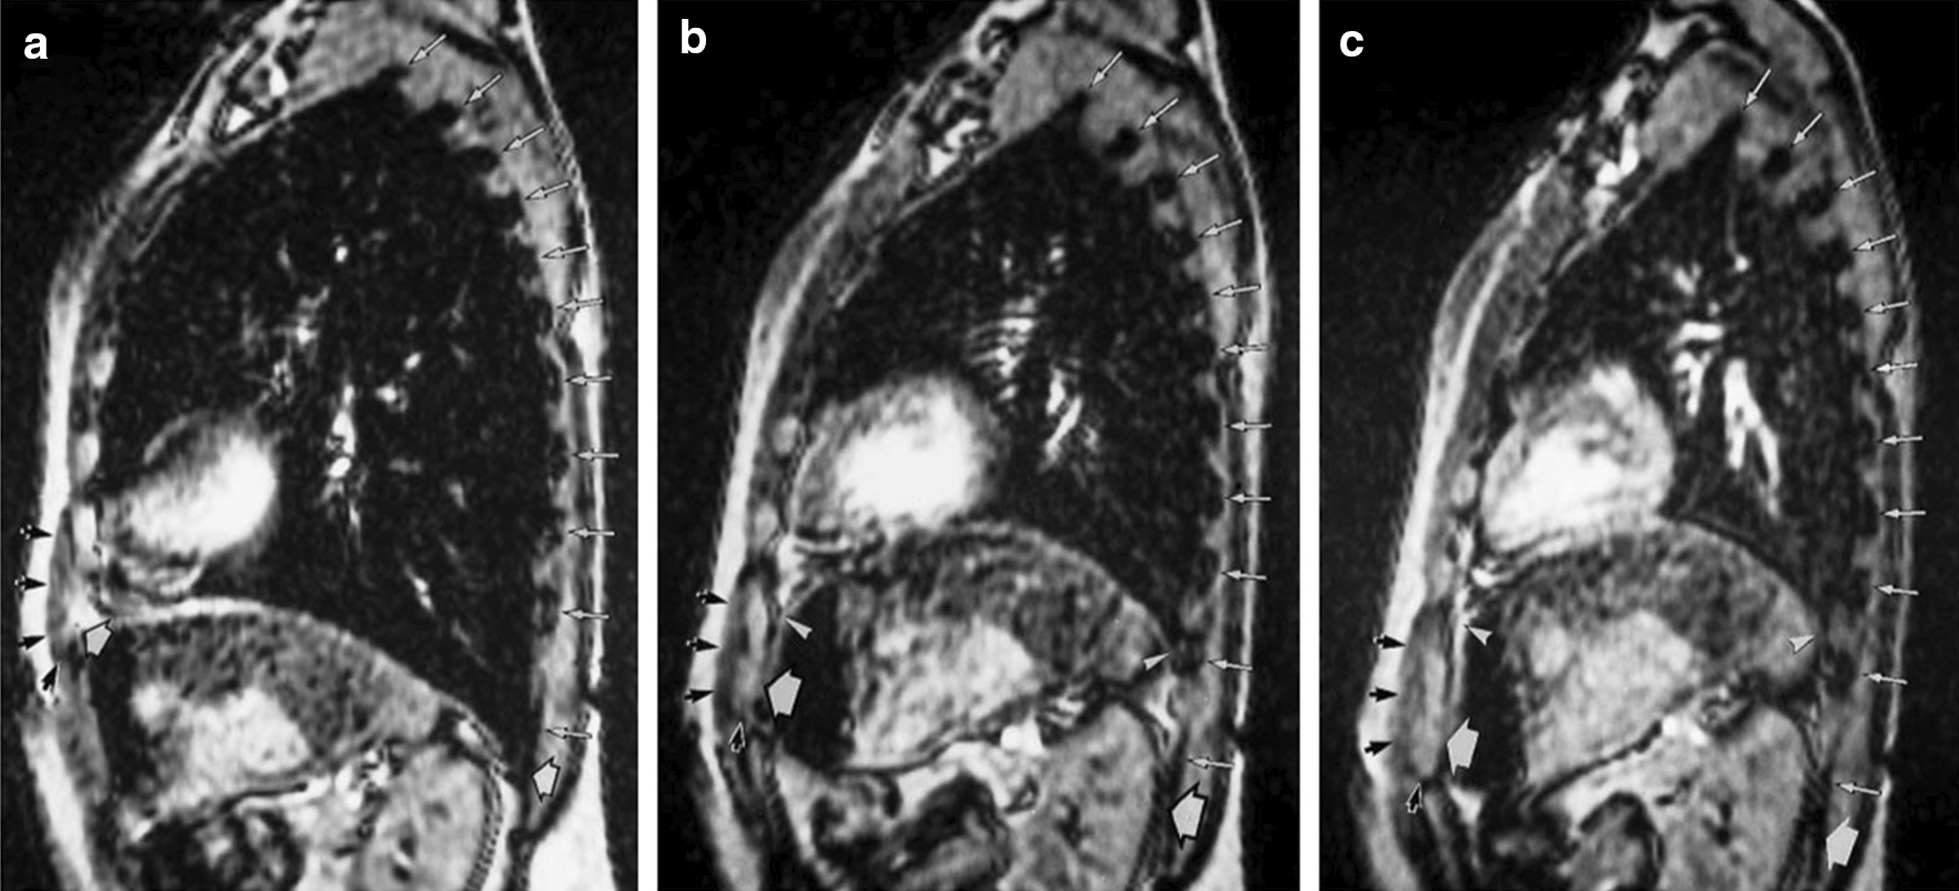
Magnetic resonance images of the diaphragm and rib cage in the sagittal view. Images were obtained at (a) total lung capacity, (b) functional residual capacity, and (c) residual volume, 7 cm to the left of the midline. At total lung capacity (a) the anterior insertion of the diaphragm onto chest wall (left white arrowhead) is in close proximity to the lower anterior ribs (black arrows) indicating that the zone of apposition is close to zero. Posteriorly, the diaphragm is positioned nearly at the level of the posterior insertion point at the twelfth rib (right white arrowhead). As the diaphragm moves to functional residual capacity (b) and residual volume (c) the zone of apposition increases indicated by the cranial movement of the upper limits of the zone of apposition (right and left thin white arrowheads). Reproduced with permission from Radiologic Society of North America: Cluzel et al. Radiology 2000;215(2):574–83
